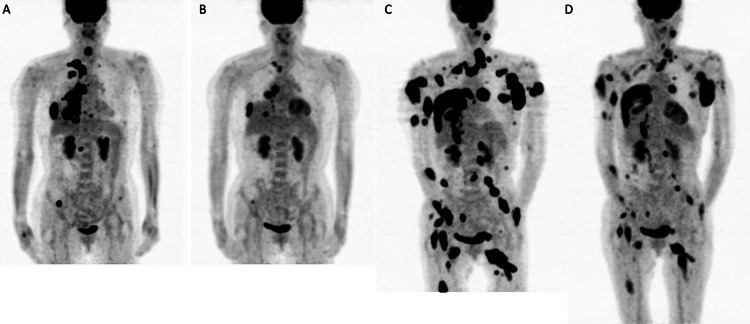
Changes in PET-CT findings during treatment. (A) PET-CT image at the time of the initial examination. (B) PET-CT image after 10 months of pemetrexed treatment. The local recurrent mass had shrunk, and the accumulations in this area, pleura, and iliac crest were all reduced. (C) PET-CT image before molecular-targeted drug treatment. 18F-Labeled fluorodeoxyglucose accumulation was observed in soft tissues, such as muscle and subcutaneous tissues, and in the left palatine tonsil. (D) PET-CT image six months after the start of molecular-targeted drug therapy. Various accumulations were reduced, suggesting that treatment was effective. PET-CT, positron emission tomography-computed tomography
